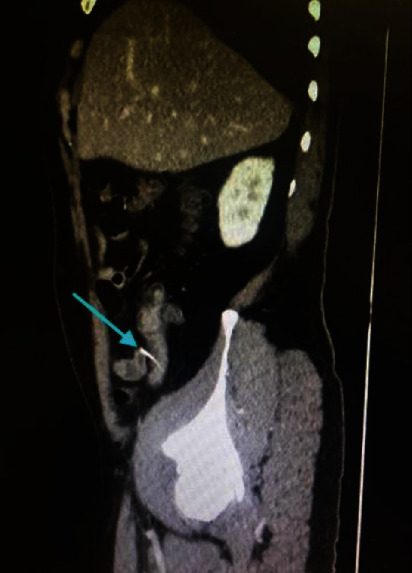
CT scan of case number 3 showing foreign body (arrow).
